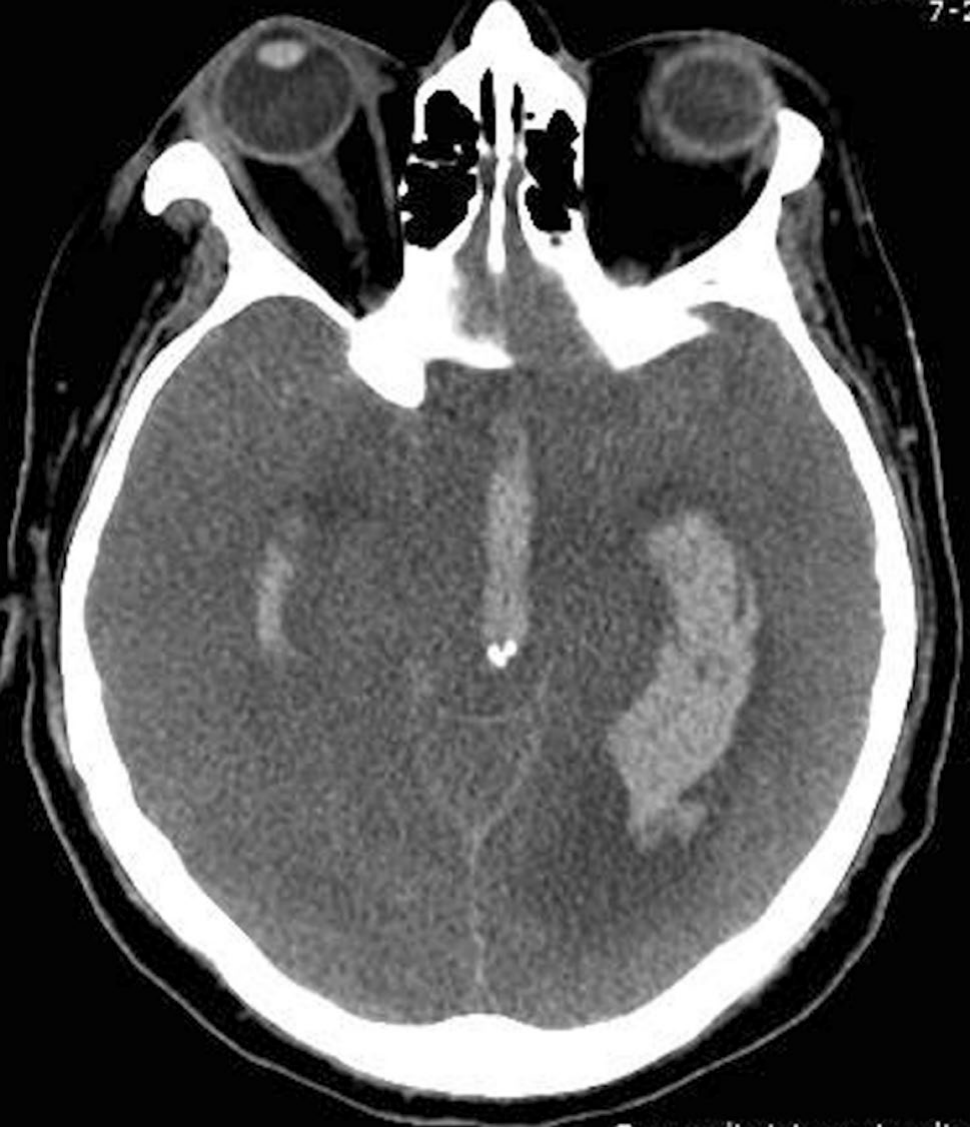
Axial CT image showing intraventricular hemorrhage in a COVID-19 patient with ECMO
